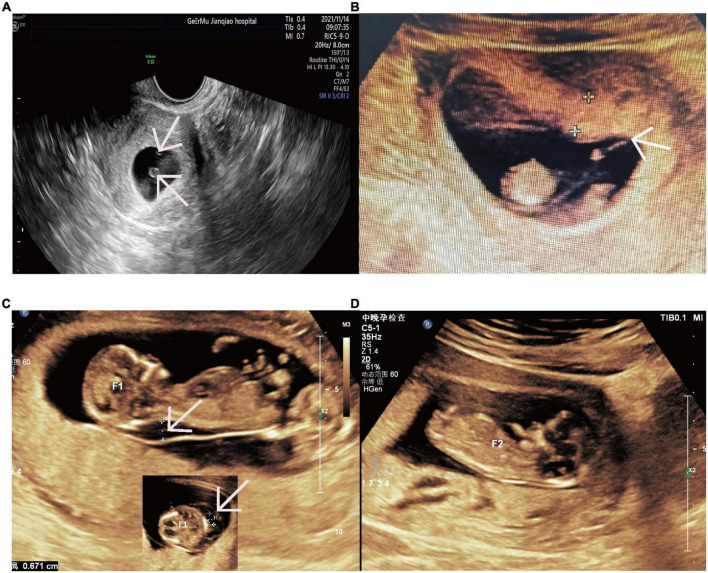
Ultrasound images revealing the monochorionic twins and twin A’s nuchal cystic hygromas and twin B’s normal NT. (A) Two yolk sacs within a single gestational sac at 6^+2^ weeks. The arrow indicates two yolk sacs. (B) The monochorionic diamnitic twins with a separating membrane of T sign at 10^+3^ weeks. The arrow indicates the distinct T sign of the separating membrane between the twins. (C) Nuchal cystic hygromas of twin A at 12^+3^ weeks. The arrows indicate the nuchal cystic hygromas of twin A from longitudinal section and transverse section. (D) The normal NT of twin B at 12^+3^ weeks. NT, nuchal translucency.
